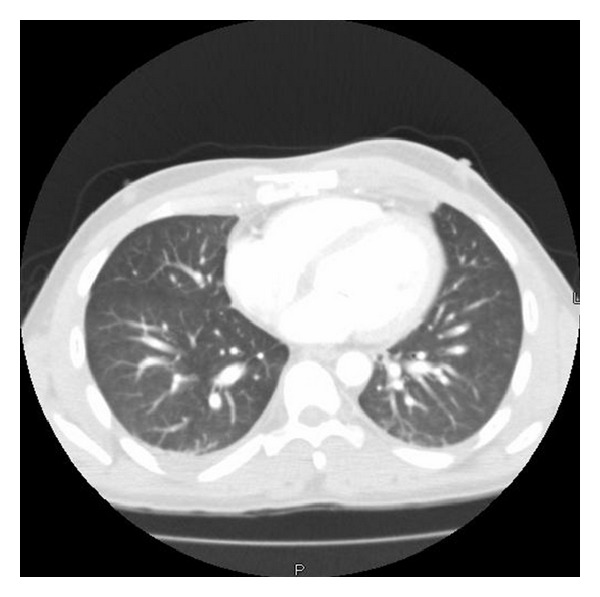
Chest CT revealing a sternal fracture and a small substernal hematoma.
